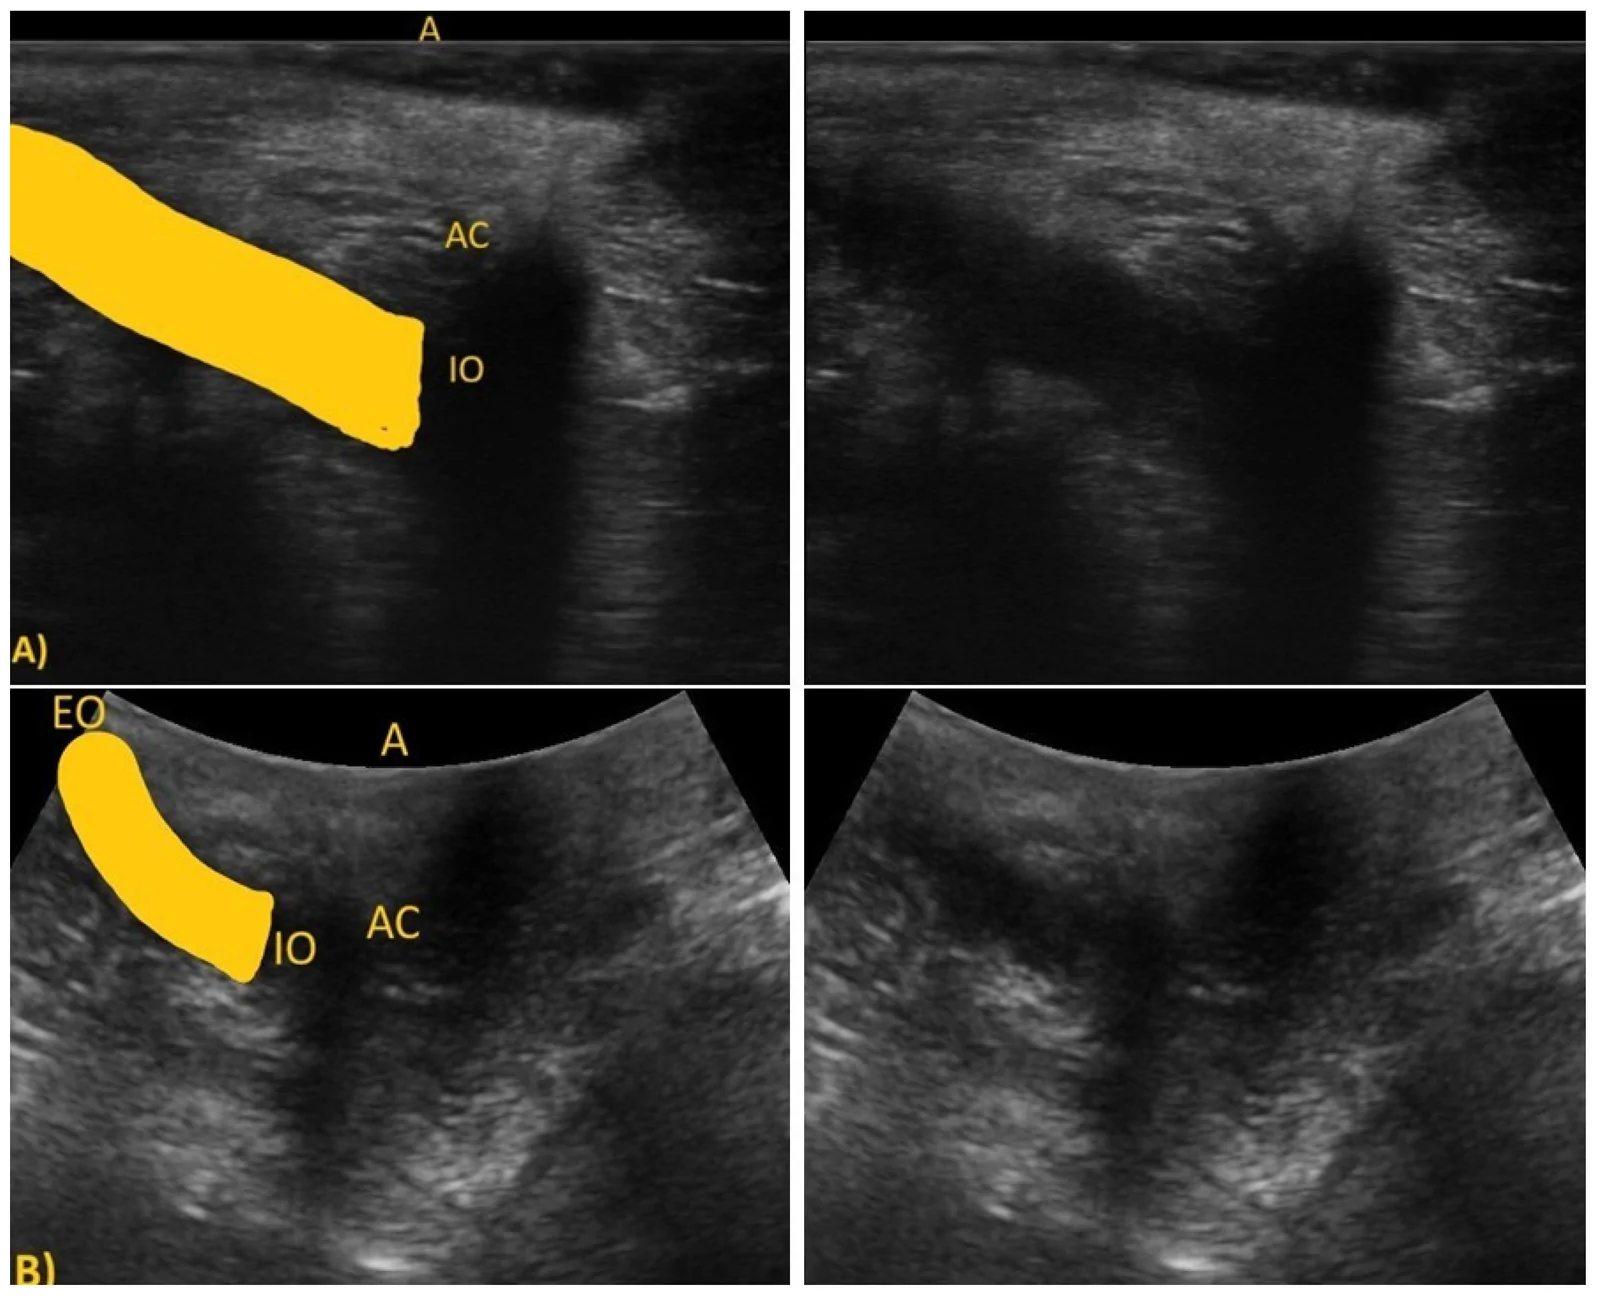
Ultrasound images: (A) were obtained using a superficial linear probe (7.5 MHz) and show a single low-type fistulous tract with obvious internal openings (IO); however, the length of the fistula makes it difficult to visualize the external opening (EO) on the same scan. (B) was taken with a deep curved probe (3.5 MHz) and shows the curved fistulous tract of 5 cm long with obvious internal openings (IO) into the anal canal (AC) located 21 mm away from the anal margin (A). The left images show the fistulous tract (thick yellow line), IO, and EO. The right images are corresponding unannotated scans.
